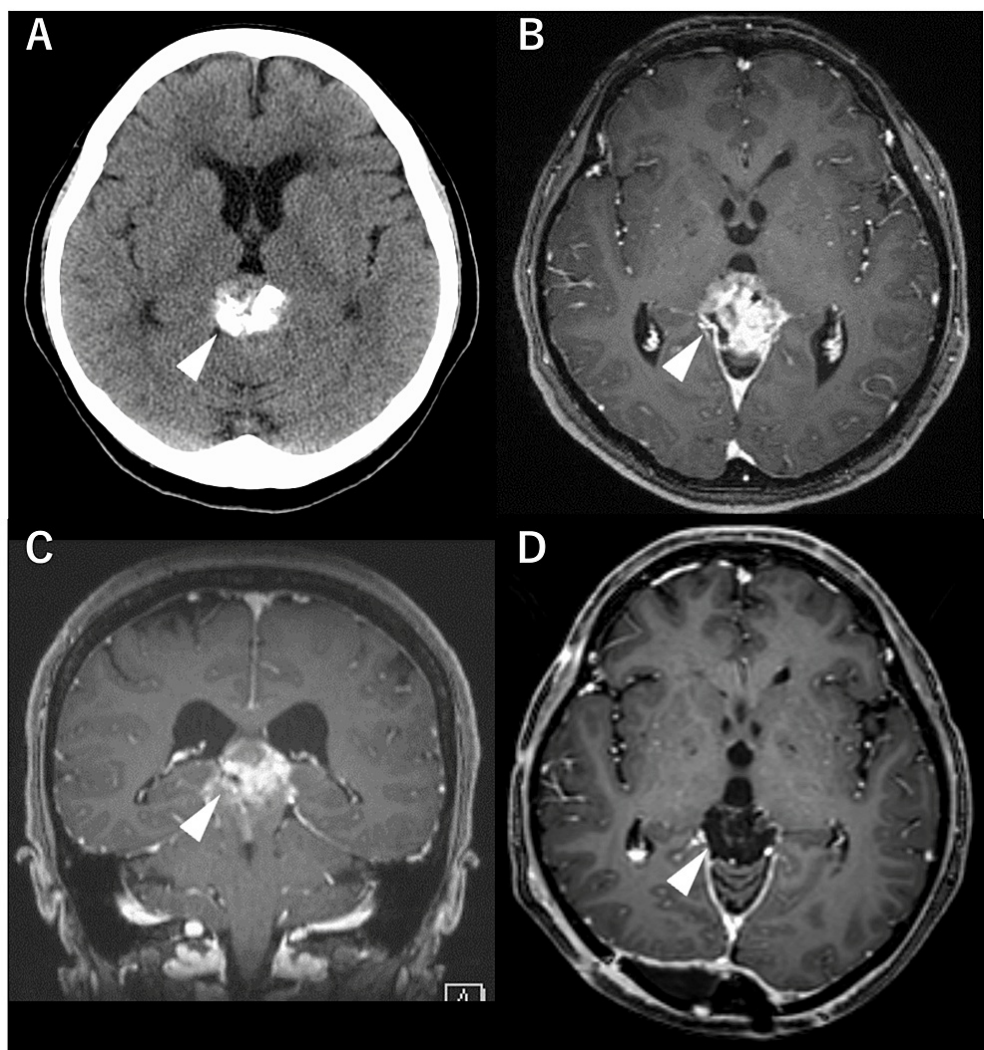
Preoperative and postoperative images Preoperative CT (A) shows a mass lesion with calcification in the pineal region (arrowhead). The mass is well enhanced on axial (B) and coronal (C) gadolinium (Gd) T1-weighted MRI images. Postoperative MRI (D) shows no residual tumor.
